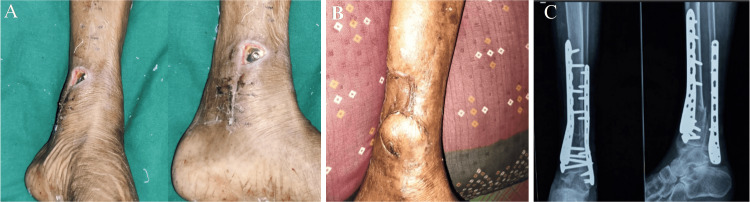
Case 4 dual-incision approach (A) A 53-year-old female was managed with a dual-incision approach (MIPO tibia and ORIF fibula), showing deep skin necrosis and implant exposure. (B) She was treated with flap coverage by plastic surgery, which eventually healed. (C) Radiograph taken two months postoperatively. MIPO: minimally invasive plate osteosynthesis, ORIF: open reduction and internal fixation
